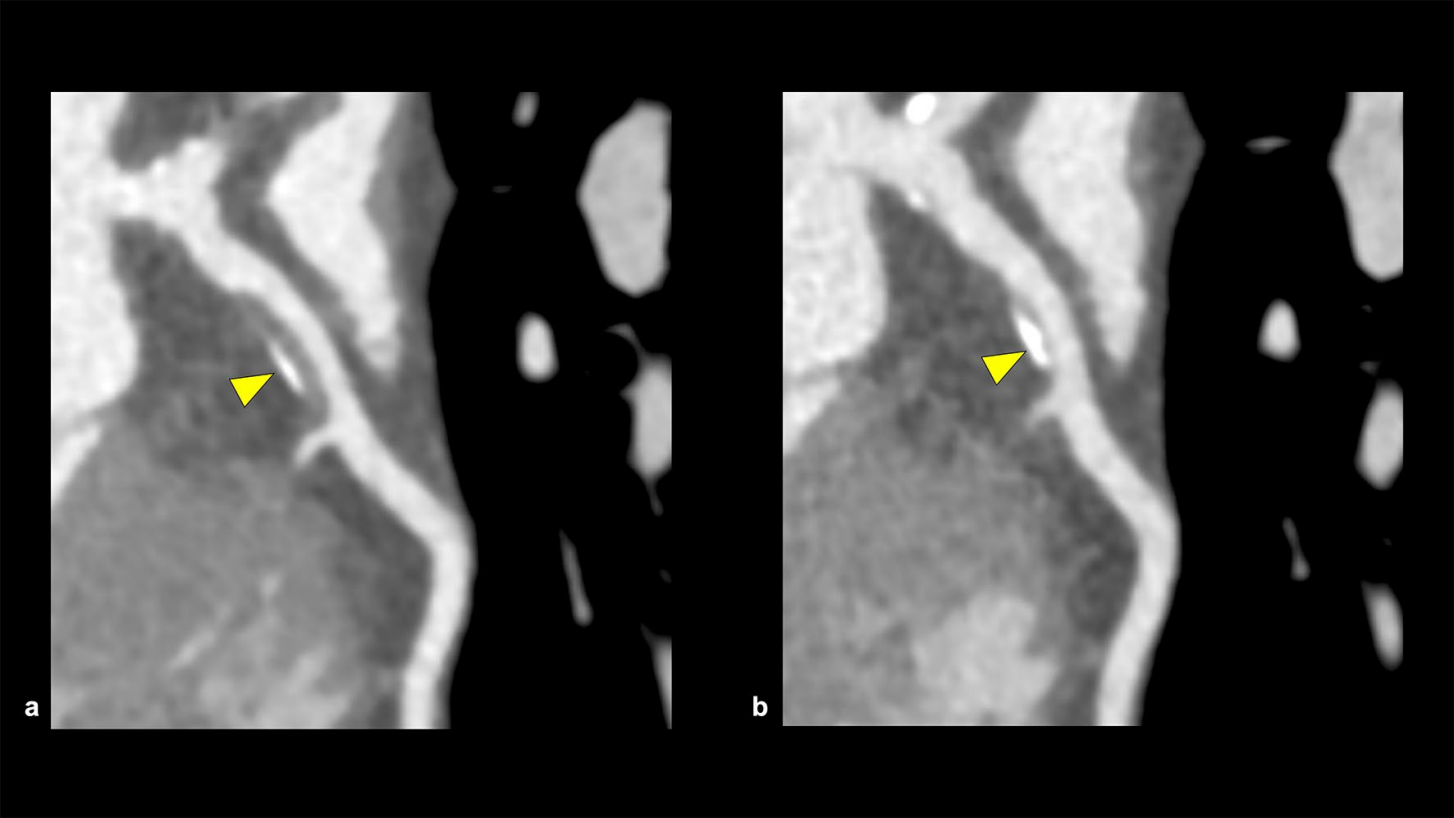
Characteristics change in coronary artery plaque on CCTA. A CPR image shows a partially calcified plaque in the proximal portion of the LAD in the first CCTA (a: arrowhead). After 3 years of optimal medication therapy, the coronary plaque was downsized and the calcified portion increased in the second CCTA (b: arrowhead). *CPR* curved planar reformation, *LAD* left anterior descending artery, *CCTA* coronary computed tomography angiography
